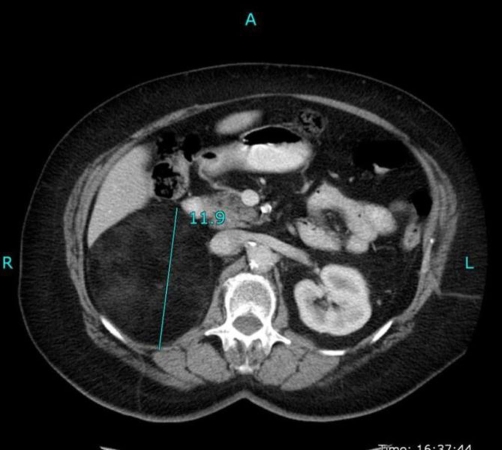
CT showing a 12 cm mass, sharply outlined, consisting of mostly fatty tissue, originating from the right kidney or the right adrenal gland.
